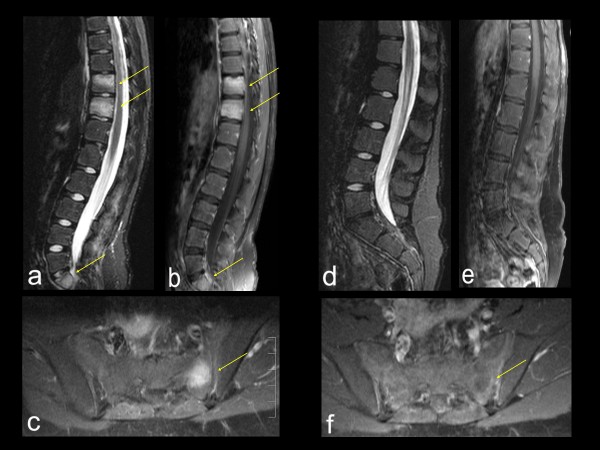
Imaging data of spinal and sacral CRMO lesions in a 10-year old girl. (A-C). Pre-treatment sagital (A and B) and axial (C) MRI. (A) STIR sequence and (B and C) post-gadolinium T1-weighted sequence reveal abnormal signal in vertebral bodies of T10, T11, and S1 (arrows), as well as in sacral ala (arrow). (D-F). Post-treatment (5 months after initiation of pamidronate) MRI using the same technique as (A-C): Complete resolution of the previously seen abnormal signal.
